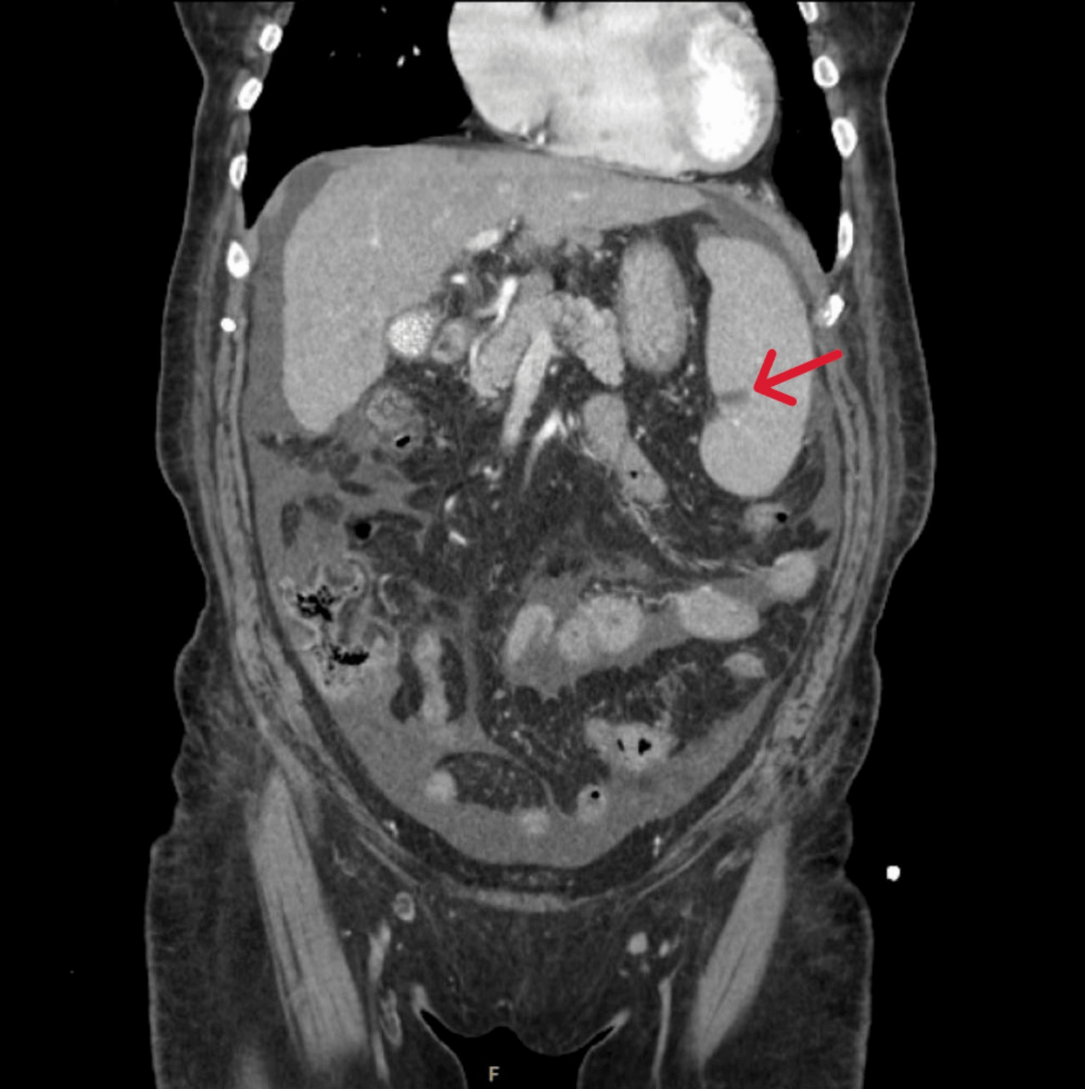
Contrast-enhanced computed tomography scan of the abdomen and pelvis Imaging revealed a small, nodular liver contour consistent with cirrhosis, mild splenomegaly measuring 13.33 cm with a focal infarct (red arrow), and minimal ascites. Although not visualized in this image, the portal, hepatic, and splenic veins were noted to be patent. The portal vein measured 13.8 mm in diameter. Features of portal hypertension were present, including large esophageal varices measuring 4.8 mm (not visualized in this image). Given the clinical context of acute myeloid leukemia, the splenic infarction is more likely attributable to leukemic infiltration and impaired splenic perfusion secondary to circulating leukemic blasts, rather than portal hypertension.
